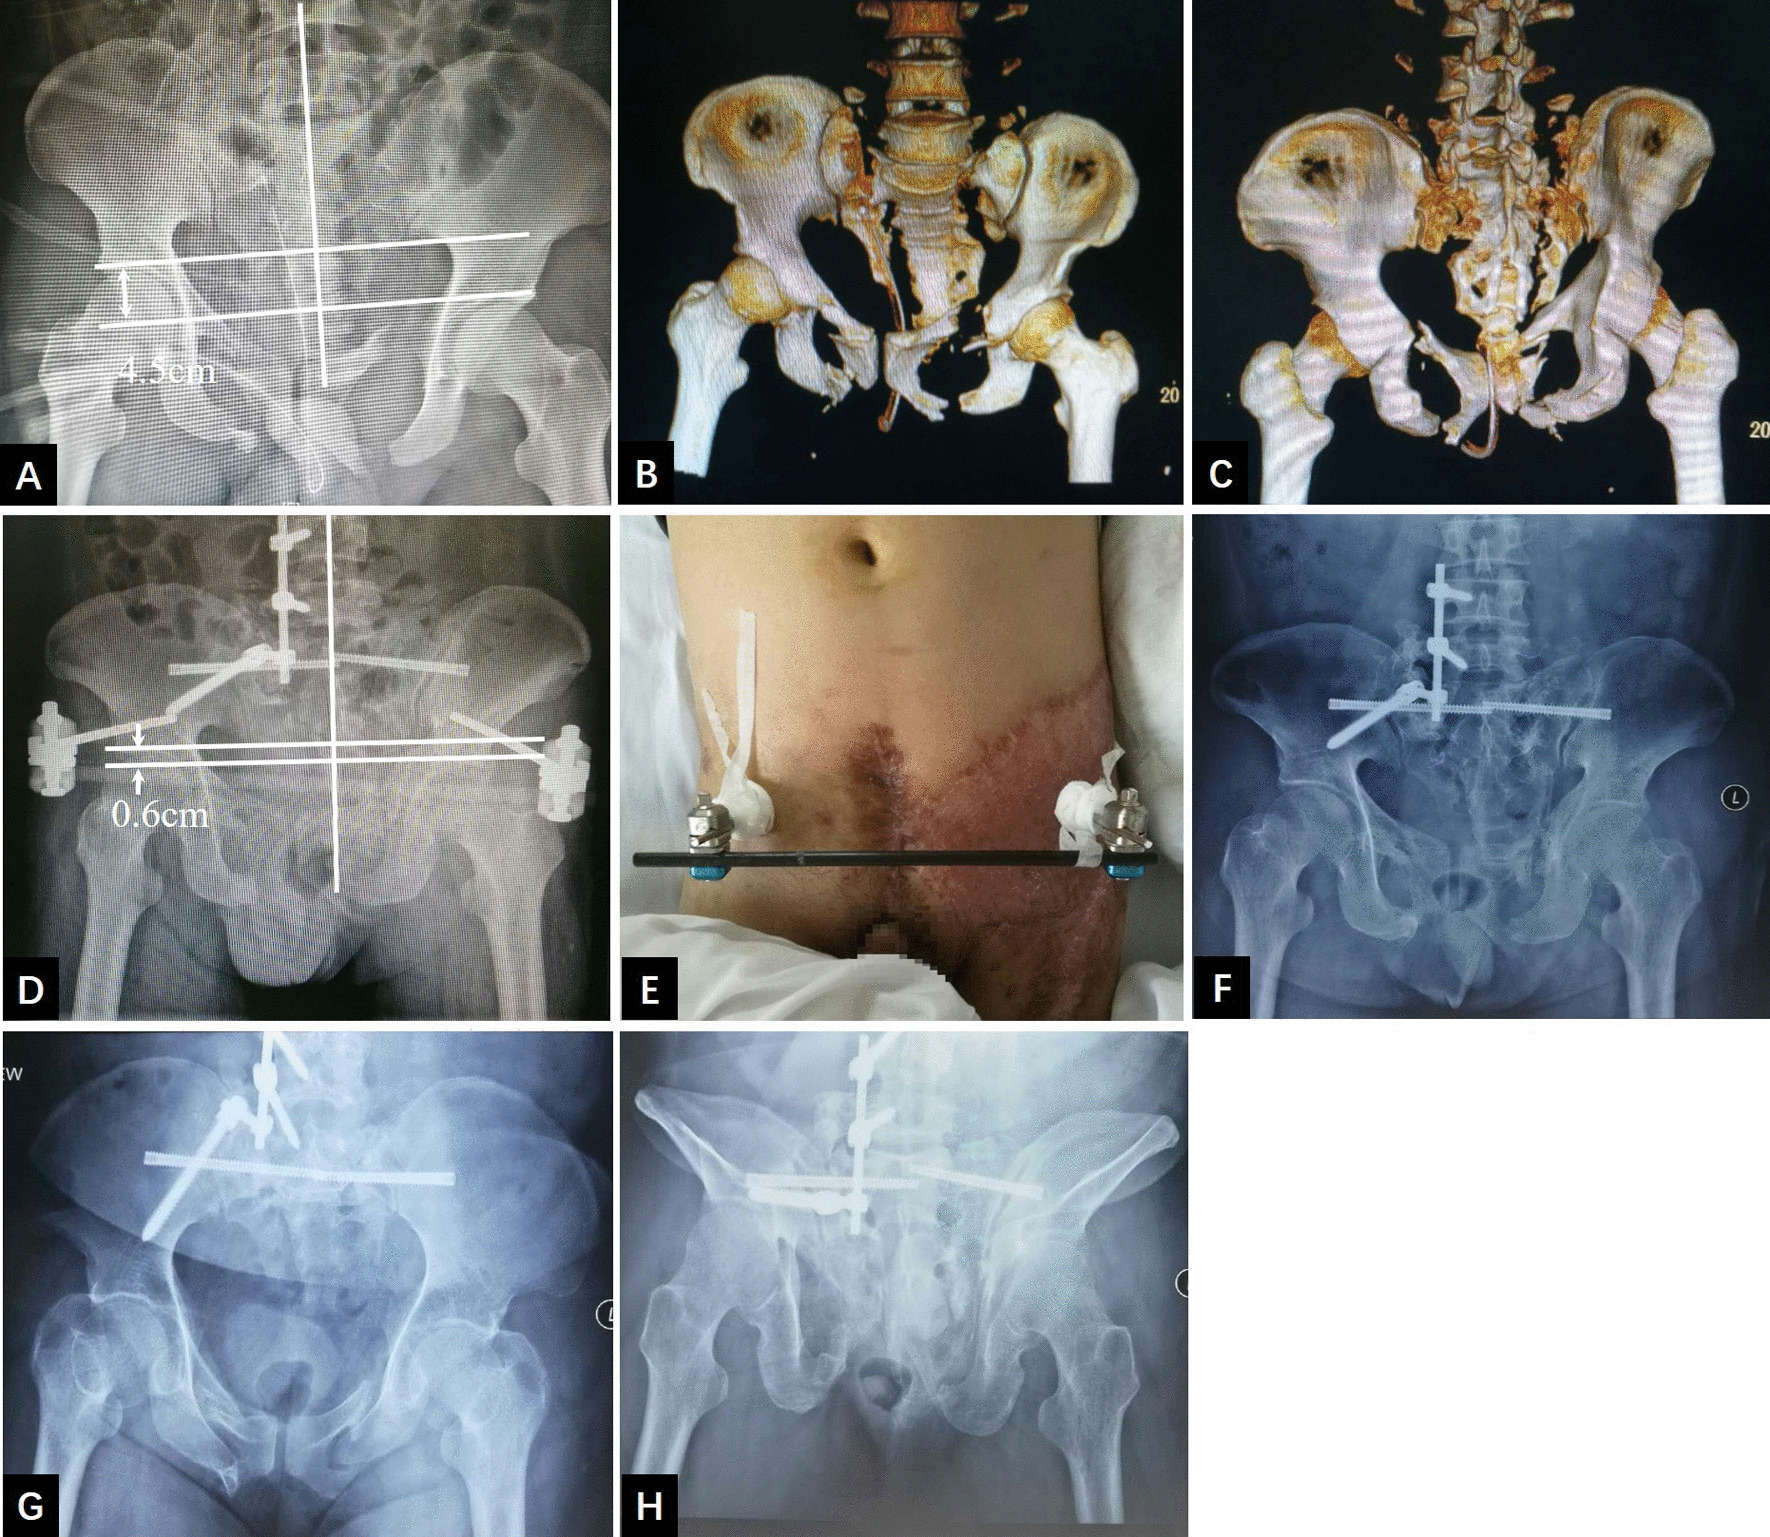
A 44-year-old male patient with a type C3.3 pelvic fracture. a–c Preoperative AP X-ray and three-dimensional CT showing significant vertical displacement (4.5 cm) of the right hemipelvis. d Osteotomy of the right sacrum, followed by lumbopelvic distraction osteosynthesis, bone grafting, and bilateral sacroiliac screw fixation, resulting in a residual unilateral vertical pelvic displacement of 0.6 cm. e Nonunion of the pubic rami combined with severe third-degree burn scars in the left inguinal region necessitating, external fixation of the anterior pelvic ring. f–h AP, inlet, and outlet X-ray at the six-month follow-up showing no displacement of the fracture (the external fixator of the anterior pelvic ring was removed 3 months after surgery)
